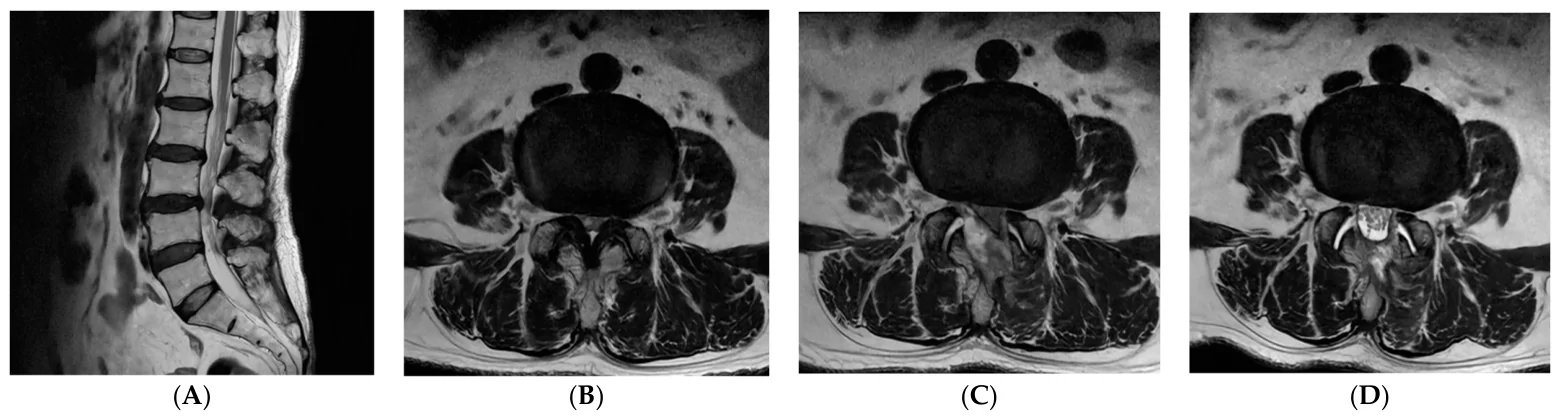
Symptomatic postoperative spinal epidural hematoma (PSEH) after L3-4 unilateral biportal endoscopic lumbar decompression. A 79-year-old female patient underwent L3-4 unilateral laminectomy and bilateral decompression for central stenosis and bilateral lateral recess stenosis. (A) Preoperative T2-weighted sagittal MRI. (B) Preoperative T2-weighted axial MRI. (C) T2-weighted axial MRI on postoperative day 2 showing grade 3 PSEH. (D) T2-weighted axial MRI after revision surgery.
